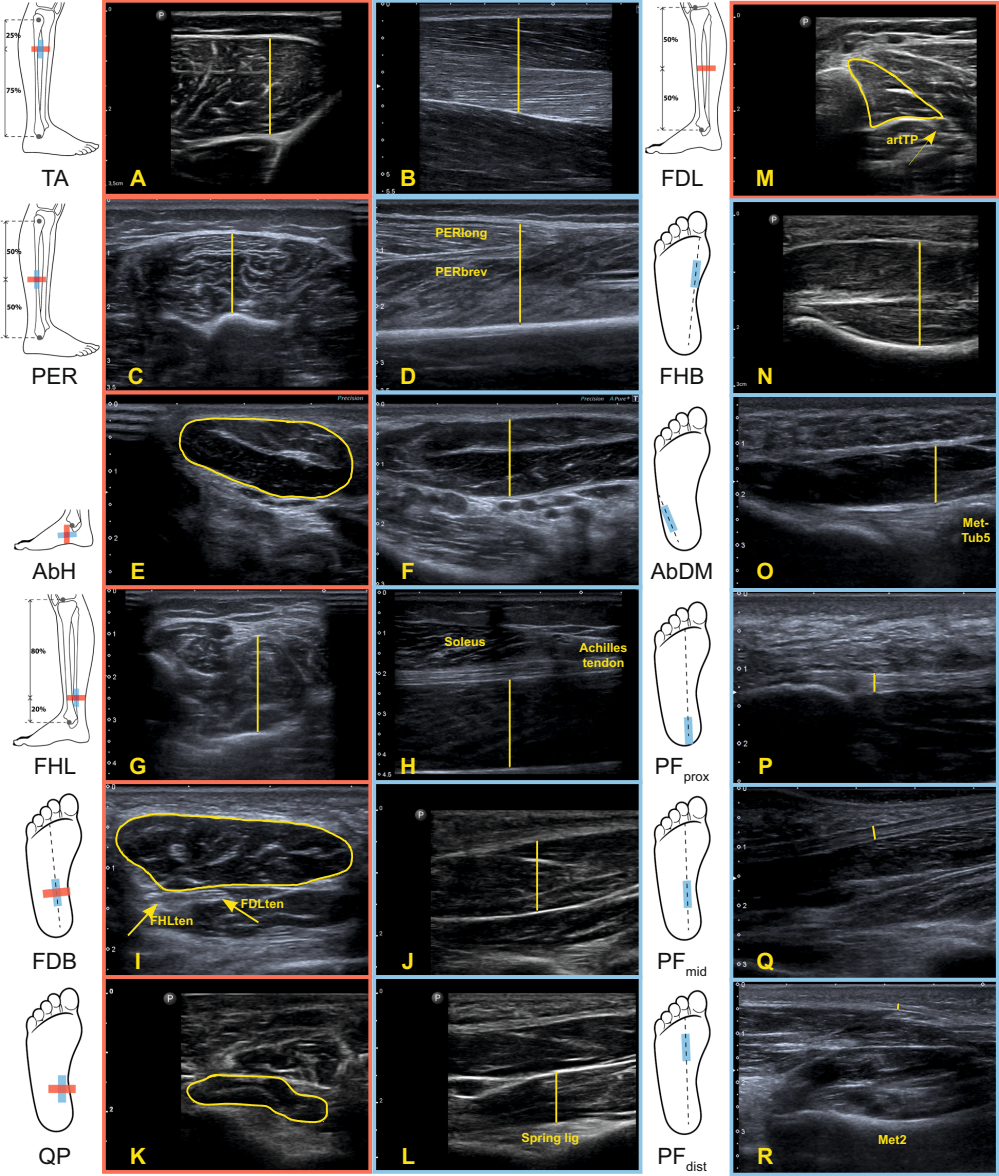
Probe position and representative ultrasound appearance for transverse (red) and longitudinal (blue) images of the selected foot muscles and plantar fascia. Ultrasound images shown are obtained with both the tablet-based (A, J-N) and the mainframe machine (B-I, O-R). TA: m. tibialis anterior, PER: m. peroneus brevis (brev) + longus (long), AbH: m. abductor hallucis, FHL: m. flexor hallucis longus, FDB: m. flexor digitorum brevis, QP: m. quadratus plantae, FDL: m. flexor digitorum longus, FHB: m. flexor hallucis brevis, AbDM: m. abductor digiti minimi, PF: plantar fascia, ten: tendon, lig: ligament, art: artery, MetTub5: 5th metatarsal tuberosity, Met2: 2nd metatarsal bone
